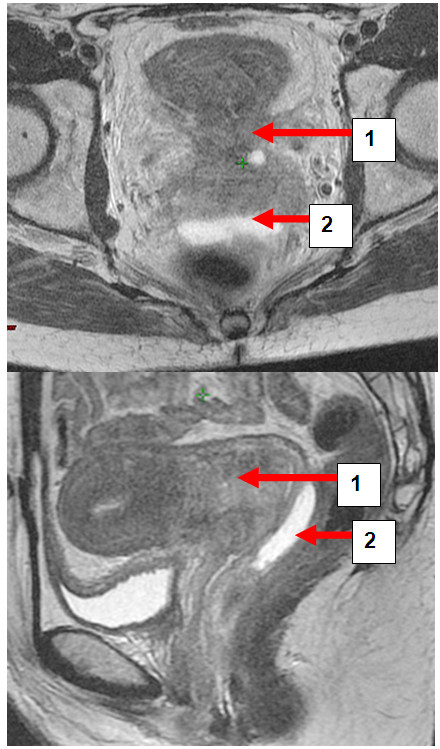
a and b: Patient LC. Transversal and sagittal pelvic MRI (T2-FSE) with cervical carcinoma (1) and rectal separation (2).
